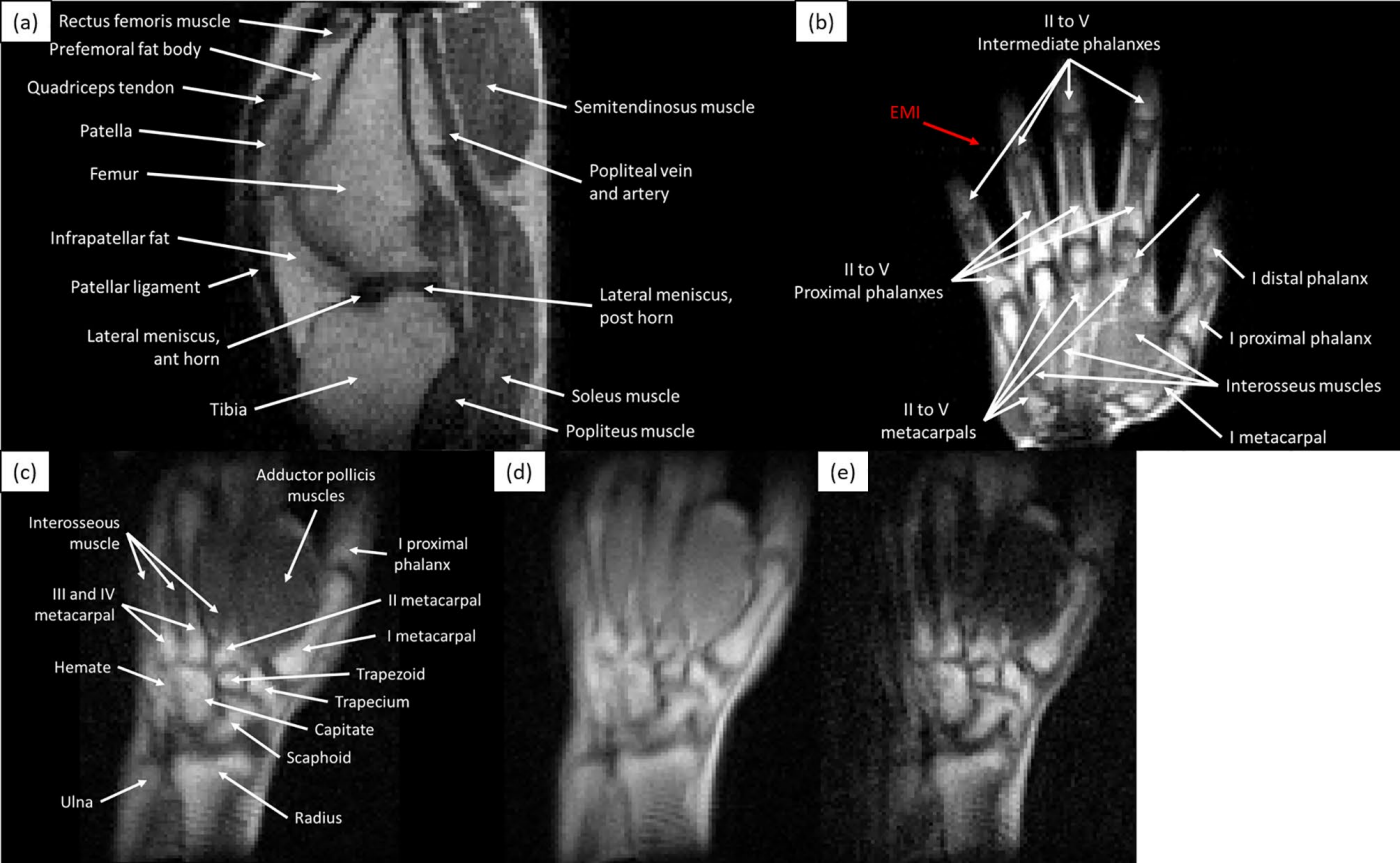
Single slices of 3D-RARE in vivo acquisitions of different volunteers in the MRI physics laboratory: (a) $$T_1$$-weighted image of a knee, acquired in 19 min; (b) $$T_1$$-weighted image of a hand (10 min), with a faint EMI line visible along the phase-encoded direction; (c)–(e) $$T_1$$, $$\rho $$ and $$T_2$$-weighted images of a wrist (12 min).
